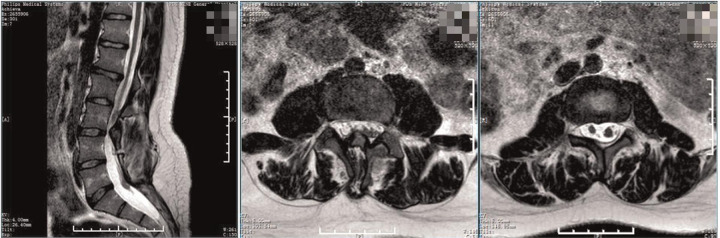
Preoperative MRI. The spinal cord was shifted down, approximately at L4 segment, with bifurcation of the lower part. The lamina of L3 segment was not fused, and the spinous process protruded into the spinal canal, resulting in spinal stenosis of the related segments.
